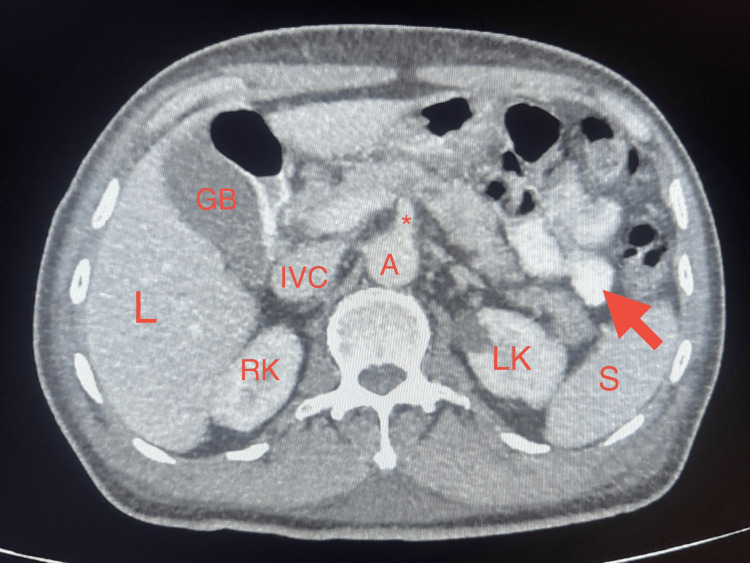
Contrast-enhanced abdominopelvic CT scan (axial abdominal view) Contrast-enhanced abdominopelvic CT scan (portal venous phase), axial view at the level of the superior mesenteric artery root, demonstrating normal distribution of vessels without evidence of intra-abdominal collection, abscess, or free fluid. The red arrow shows loops in the small intestine with contrast. GB=Gallblader, L=liver, RK: Right kidney, LK: Left kidney, IVC: Inferior vena cava, A: Abdominal aorta, *=Superior mesenteric artery, S: Spleen. Informed consent was given for the publication of the study.
